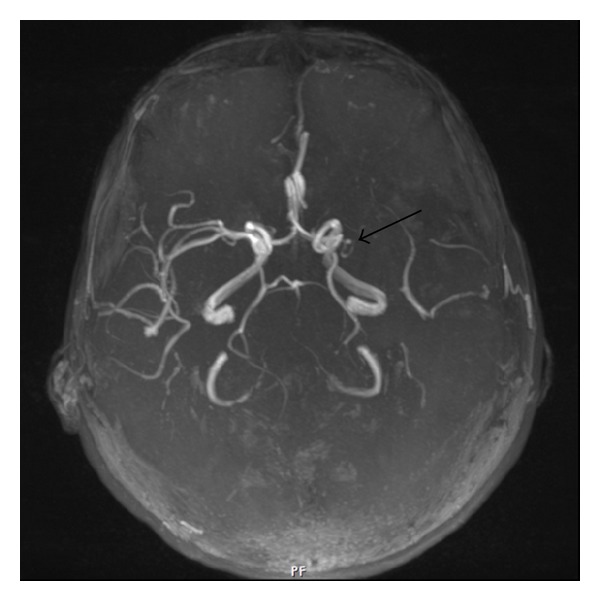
Occlusion from origin of left middle cerebral artery on MRI angiography.
